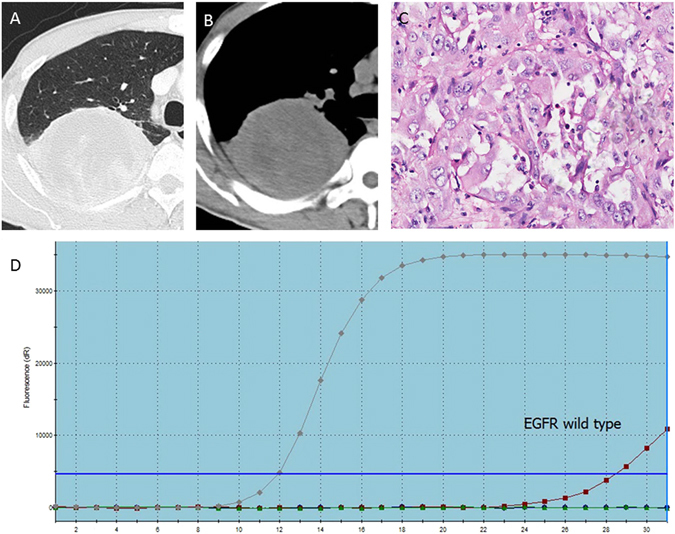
A 46-year-old man with a smoking history of 30 years had right upper lobe lung adenocarcinoma in EGFR wild type, in whom axial CT images (A,B) show a solid lump about 90 mm with a litte fibrosis and no pleural retraction or Spicule sign. The PCR picture (C) and pathological photo (D) show that the red line has no association with the upper line, indicating the EGFR wild type.
